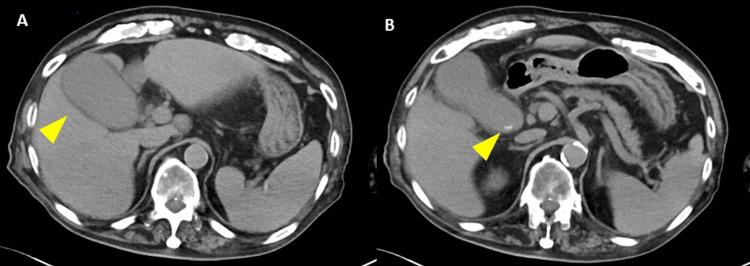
Initial non-contrast abdominal computed tomography (A) Marked distension of the gallbladder with increased density of the surrounding fat tissue. (B) A 6-mm calculus identified at the gallbladder neck.
